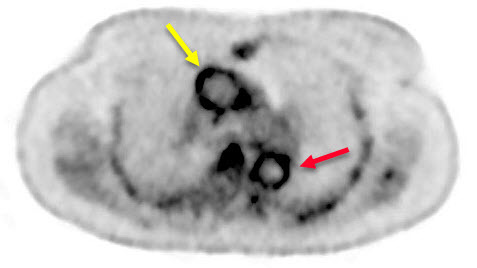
A PET-CT Image of GCA Patient with Active Vasculitis of Thoracic Aorta Showing Increased FDG Uptake in Arterial Wall of Both the Ascending (Yellow Arrow) and Descending (Red Arrow) Aorta.
